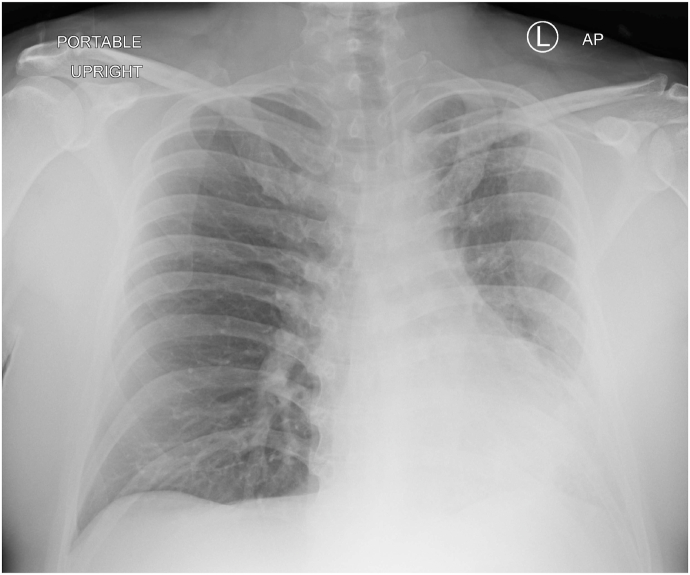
Chest X-ray showing left lung hypoplasia, left lung hyperlucency and reticulations, a hyperinflated right lung, and a left mediastinal shift.
